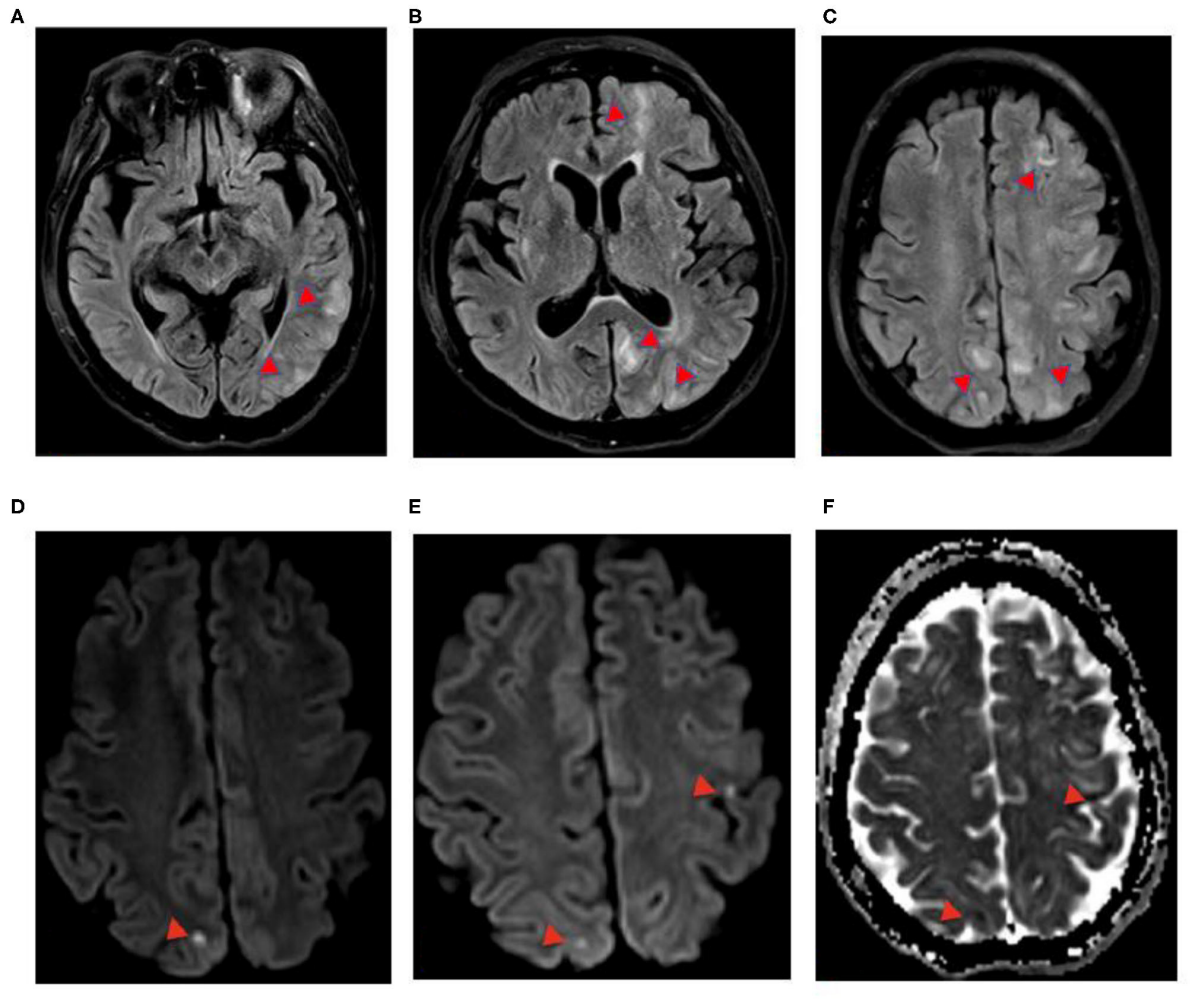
(A–F) Case 3: 64-year-old COVID-positive female, axial 3-T MRI. (A–C) Axial FLAIR. (D,E) Axial DWI. (F) Axial apparent diffusion coefficient (ADC). FLAIR hyperintensity in the left was greater than that in the right cerebral hemispheres, with punctate DWI hyperintensity (red arrowheads) with ADC restricted diffusion. (F) Left precentral sulcus and right superior medial right parietal lobe, foci of FLAIR, DWI hyperintensity without restricted diffusion, and ADC T2-shine through, concerning posterior reversible encephalopathy in a patient with elevated blood pressure.
